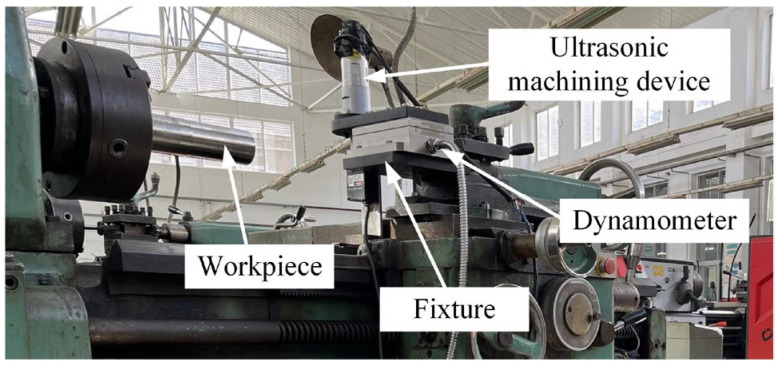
Experimental device of Ultrasonic turning.
